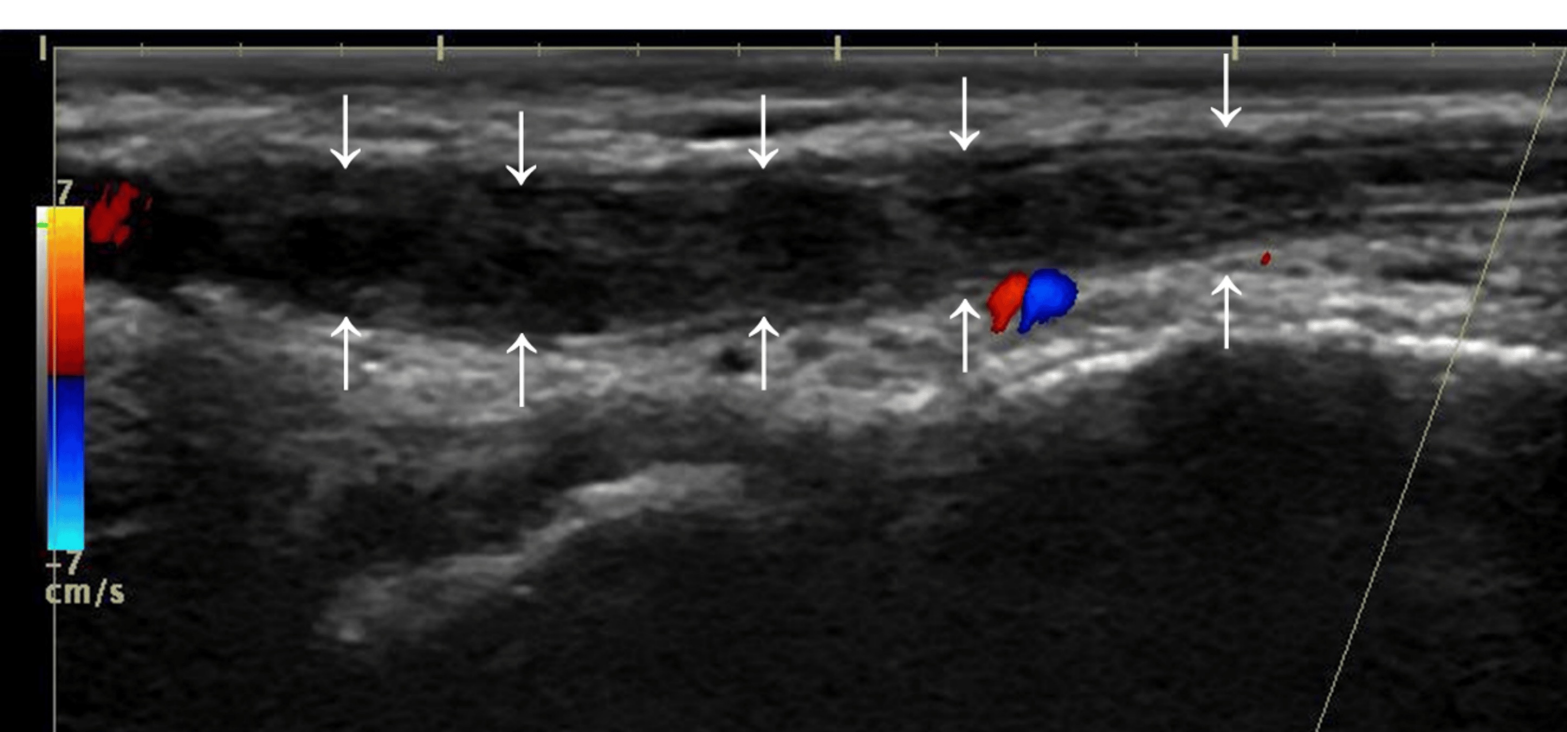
Dorsal penile vein thrombosis (arrows) detected by color Doppler ultrasonography
